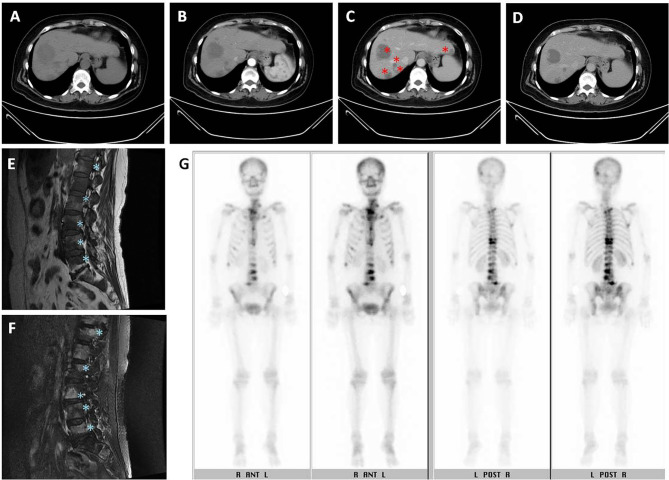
Initial imaging examination. (A–D) Dynamic abdominal computed tomography (CT): (A) multiple variable sized tumor lesions in both hepatic lobes with hypodensity in precontrast image, (B) persistent poor enhancement showed in arterial phase, (C) portal venous phase taken on November 26, 2015, original tumor lesions indicated by red asterisk symbol, and (D) delayed-postcontrast phase. (E, F) L-spine MRI showed multiple tumor lesions indicated by blue asterisk symbol. (E) Fluid attenuated inversion recovery (FLAIR) T1-weighted MRI. (F) Fast relaxation fast spin echo (FRFSE) T2-weighted MRI. (G) Tc-99m MDP whole body bone scan showed multiple increased tracer uptake in the skeletal system, compatible with multiple bone metastases.
